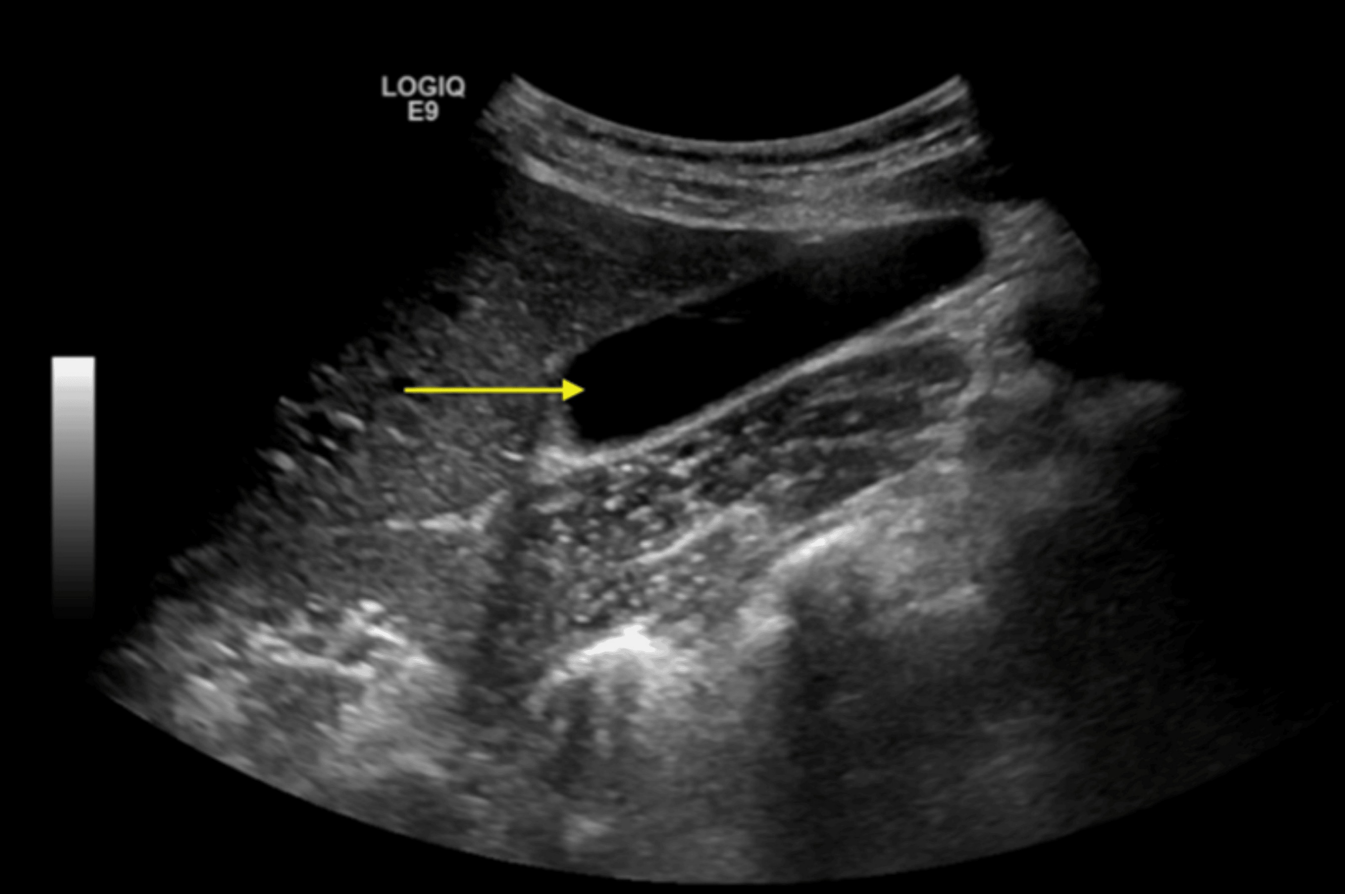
Ultrasound of the right upper quadrant (RUQ) shows a normal-appearing gallbladder without gallstones.
